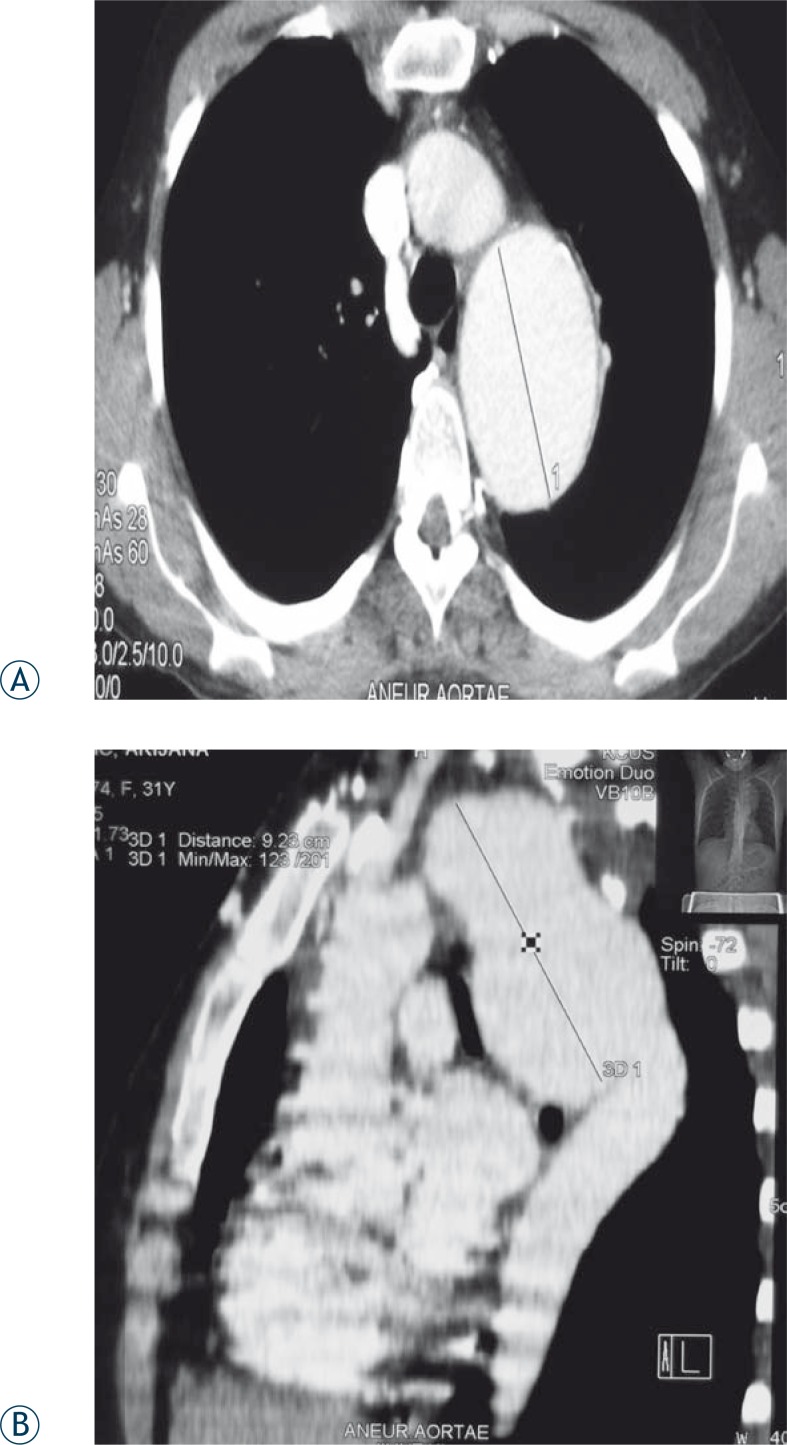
CT of posttraumatic pseudoaneurysm of the aortic isthmus.
